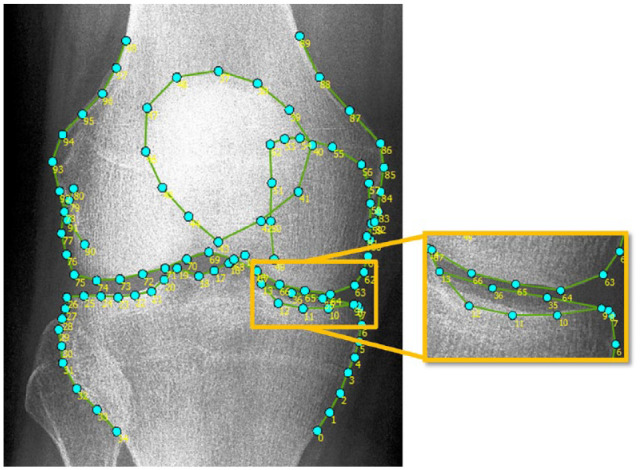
The 99-points model automatically placed on knee radiograph using BoneFinder®.^12,13^ The points on the lateral tibia plateau were placed on the anticipated anterior edge.
